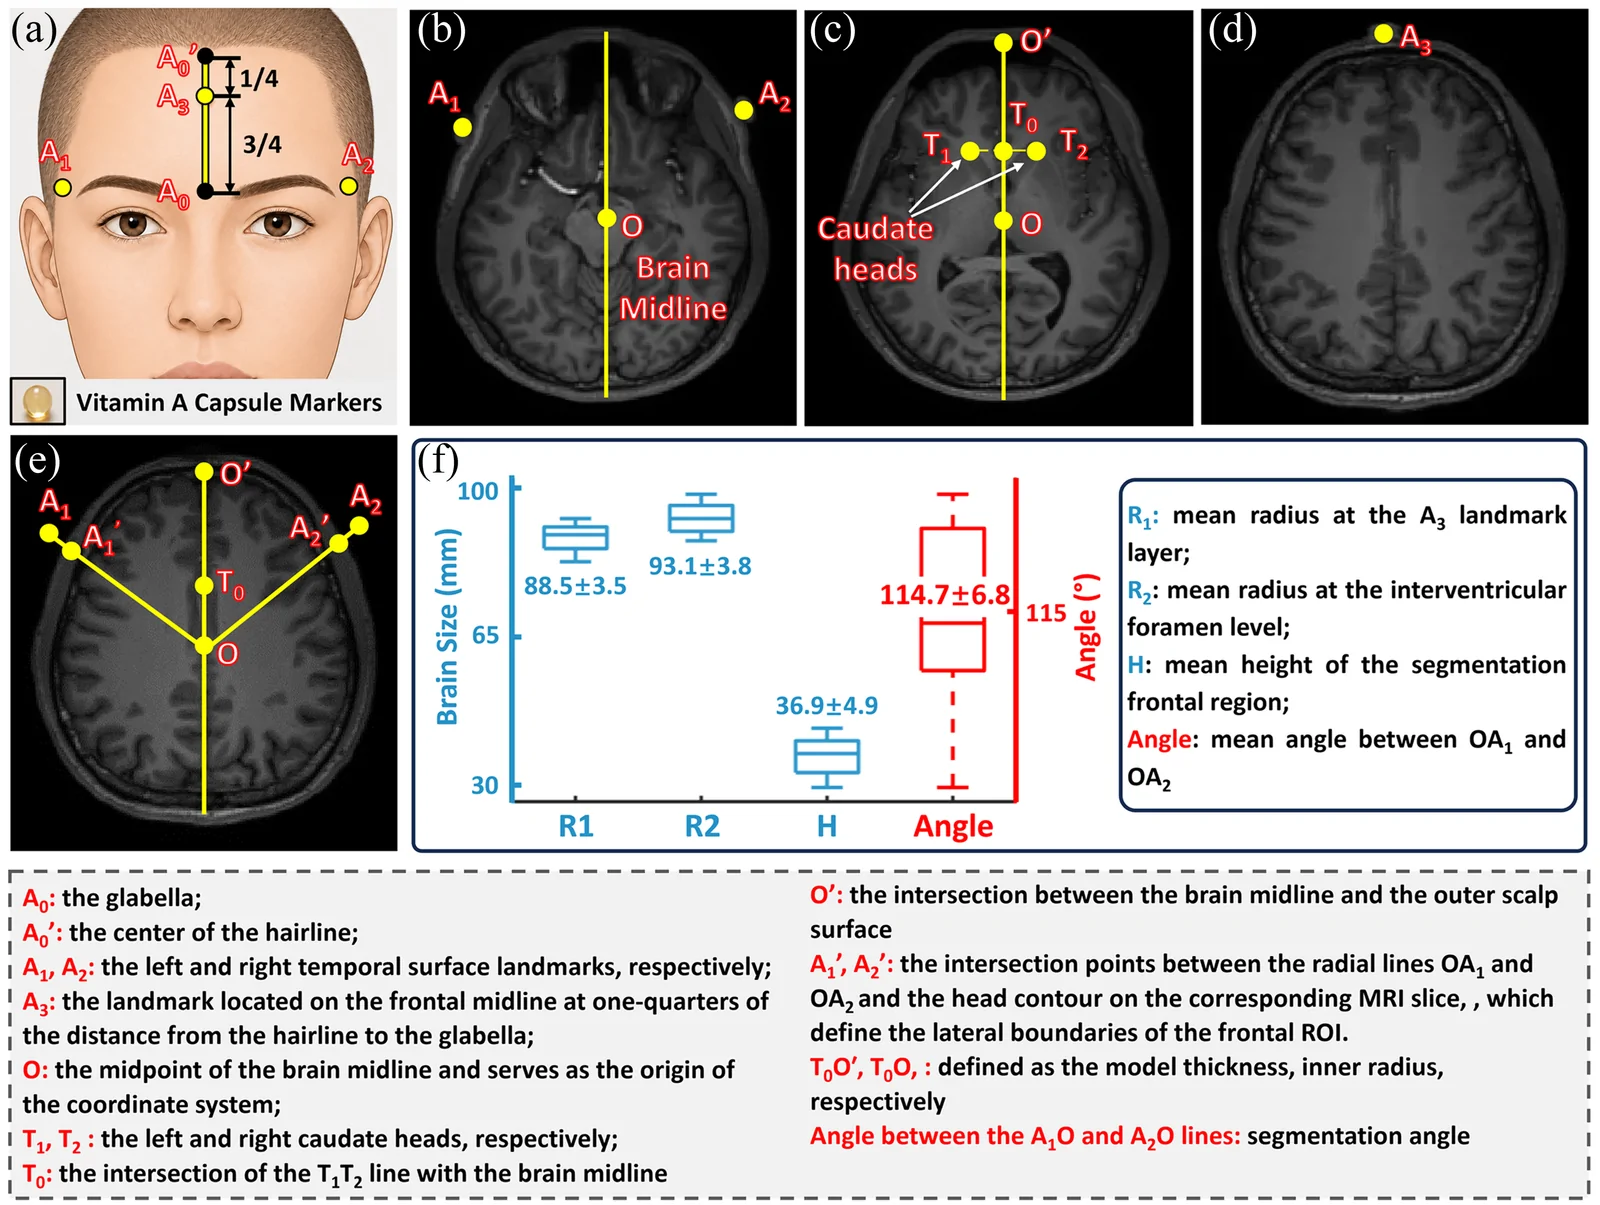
Anatomical landmarking, tissue segmentation, and head dimension statistics. (a) Placement of vitamin A capsules as MRI-visible fiducial markers at $A_{1}$, $A_{2}$ and $A_{3}$. (b) Axial slice containing markers $A_{1}$ and $A_{2}$. (c) Starting layer defined at the caudate-head level. (d) Ending layer identified by the first appearance of marker $A_{3}$. (e) Representative intermediate layer showing the definition of frontal-region boundaries and tissue segmentation. (f) Statistics of head dimensions used for standard DB model construction.
